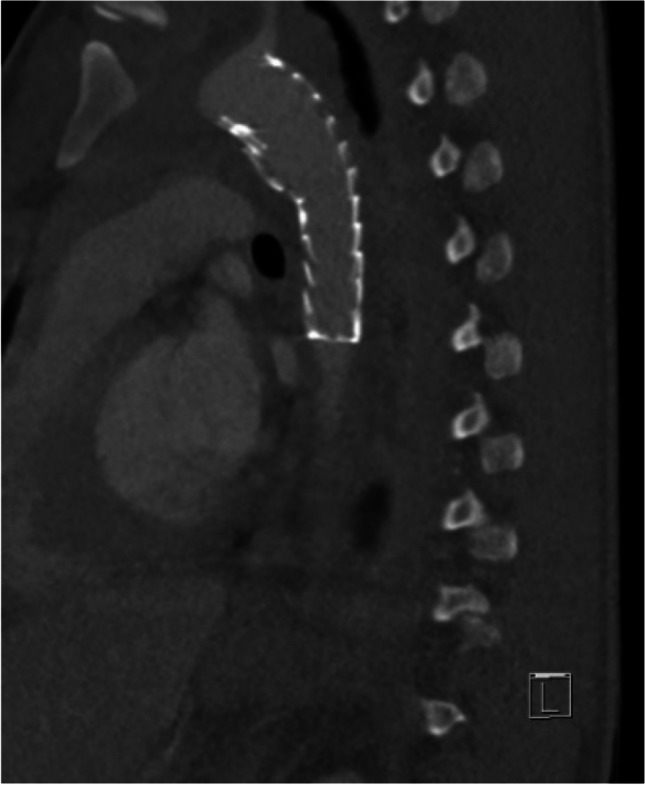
Postoperative CT-imaging after implantation of a thoracic stent
